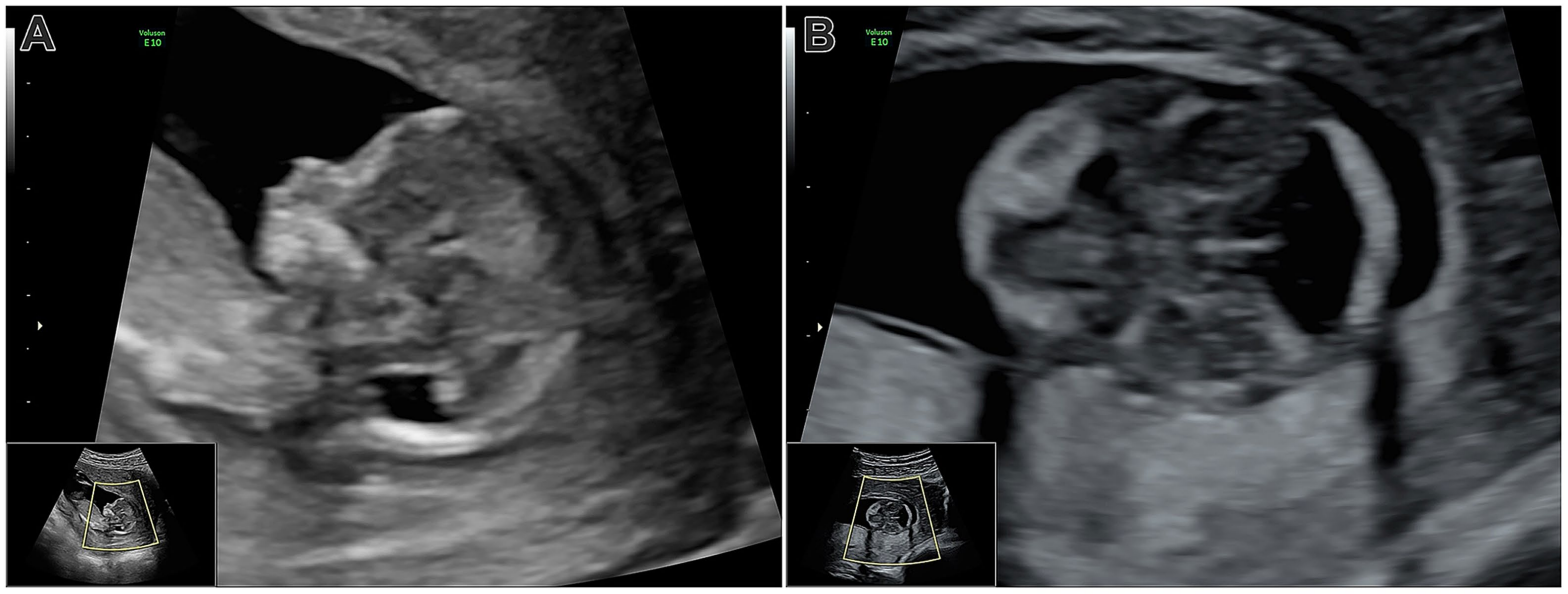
(A) Ultrasonography at week 13, mid-sagittal view: Pathological appearance of the posterior cranial fossa, showing the diencephalon and brainstem. The enlarged posterior fossa and fourth ventricle are visible, but intracranial translucency is absent. (B) Transverse view of the skull base: The posterior fossa lacks the typical parallel lines of the brainstem and choroid plexus, with fourth ventricle dilation evident.
